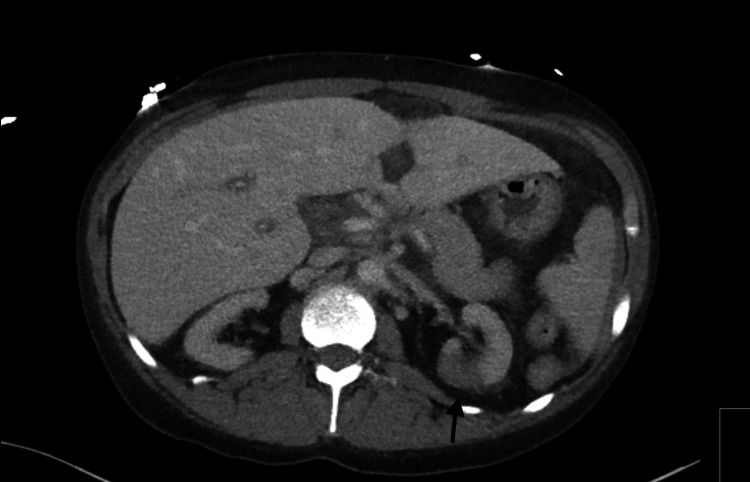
CT abdomen significant for left-sided perinephric stranding as shown by the arrow
